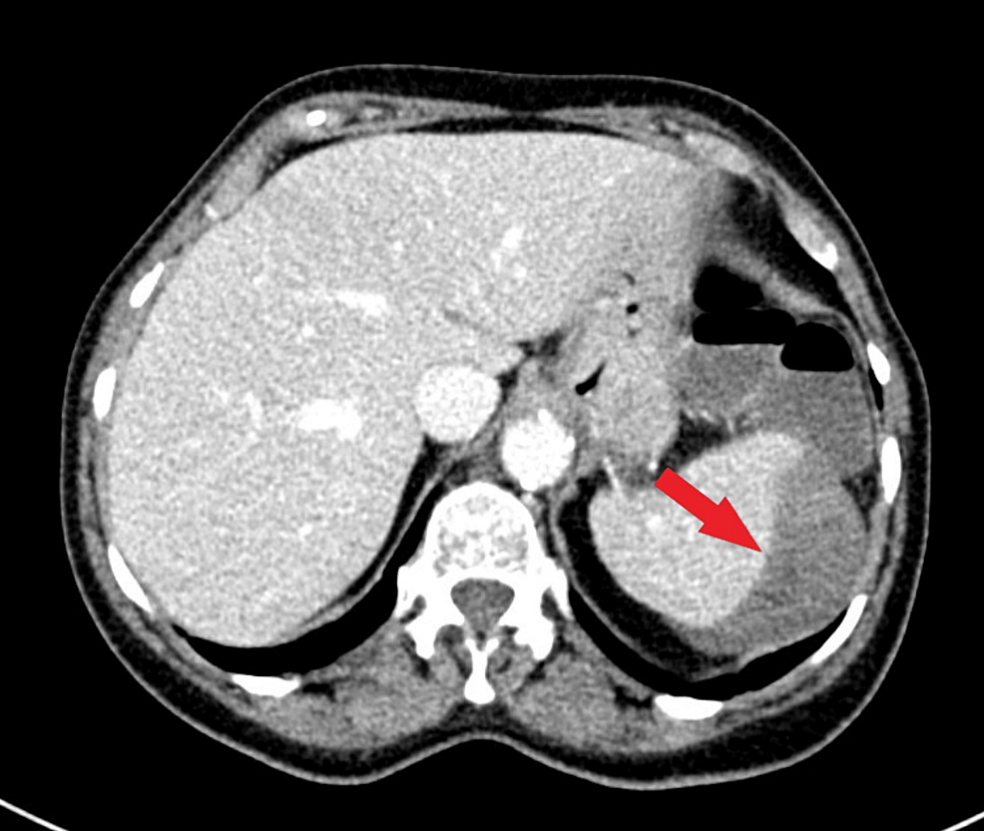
CT abdomen axial section showing subcapsular splenic hematoma (red arrow).
